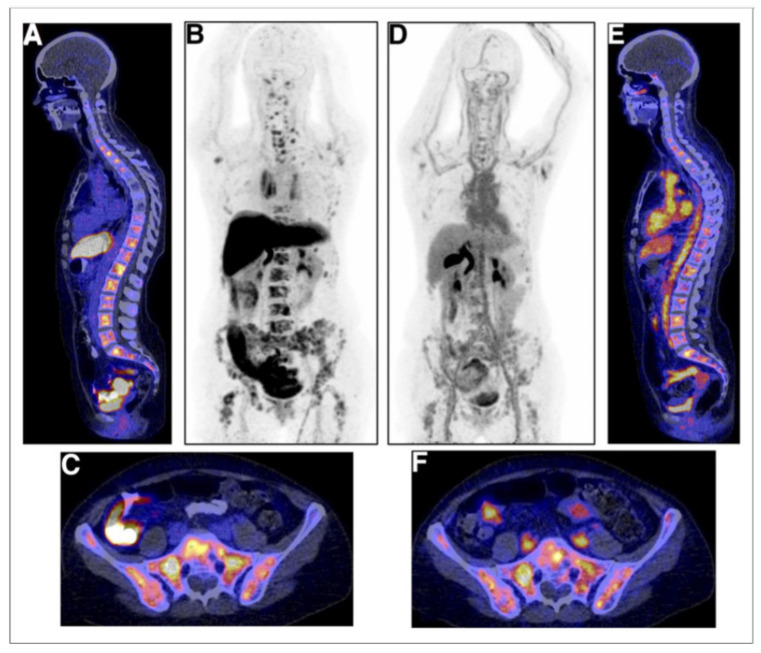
Examples of [^18^F]FES (A–C) and [^18^F]FDHT (D–F) studies in same breast cancer patient with multiple bone metastases. (A) [^18^F]FES PET/CT scan with physiological uptake in liver, small intestine, and urinary tract and pathological uptake in multiple vertebra. (B) [^18^F]FES PET MIP allow for visualization of [^18^F]FES biodistribution. (C) [^18^F]FES PET/CT with physiologic uptake in small intestine and pathological uptake throughout pelvic bones. (D) [^18^F]FDHT PET MIP, with physiologic uptake in heart and excretion through liver and urinary tract. (E) [^18^F]FDHT PET/CT with pathologic uptake in multiple vertebrae. (F) [^18^F]FES PET/CT with physiologic uptake in large vessels and small intestines and pathologic uptake throughout pelvic bones [47]. Note: [47] © by the Society of Nuclear Medicine and Molecular Imaging, Inc.
